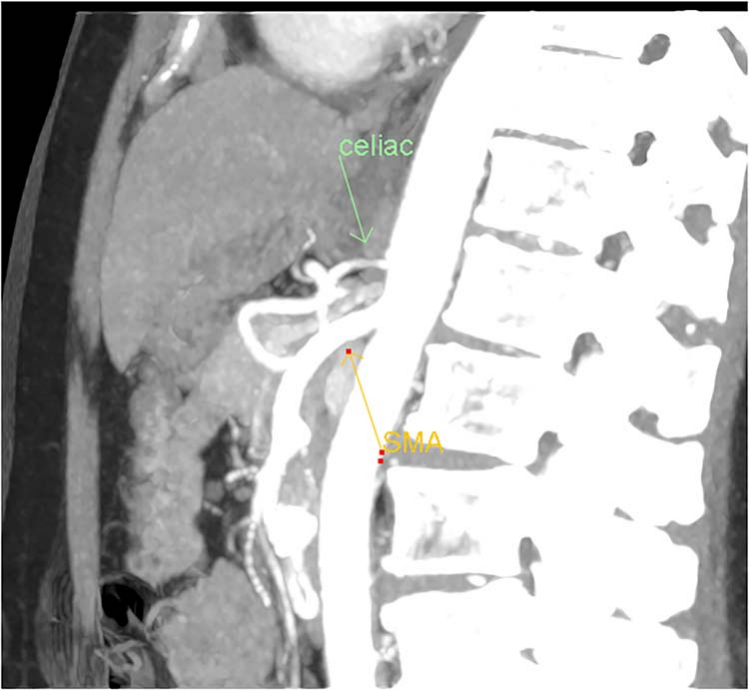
Multiphase CT pancreas, sagittal view: abnormal communication between the CA and the SMA. The celiac axis is markedly narrowed, >90%.
